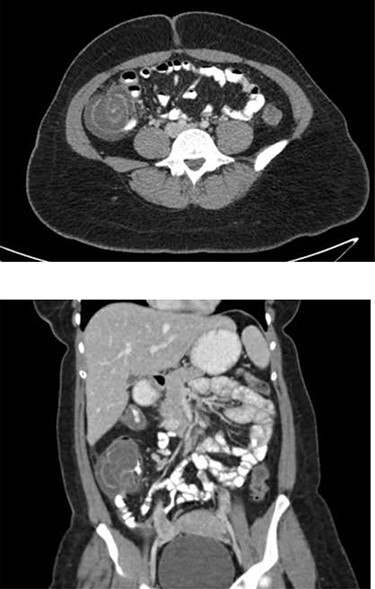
Representative axial (top) and coronal (bottom) images from patient’ s CT abdomen/pelvis with PO and IV contrast demonstrating cecal thickening and ileocolic intussusception.
